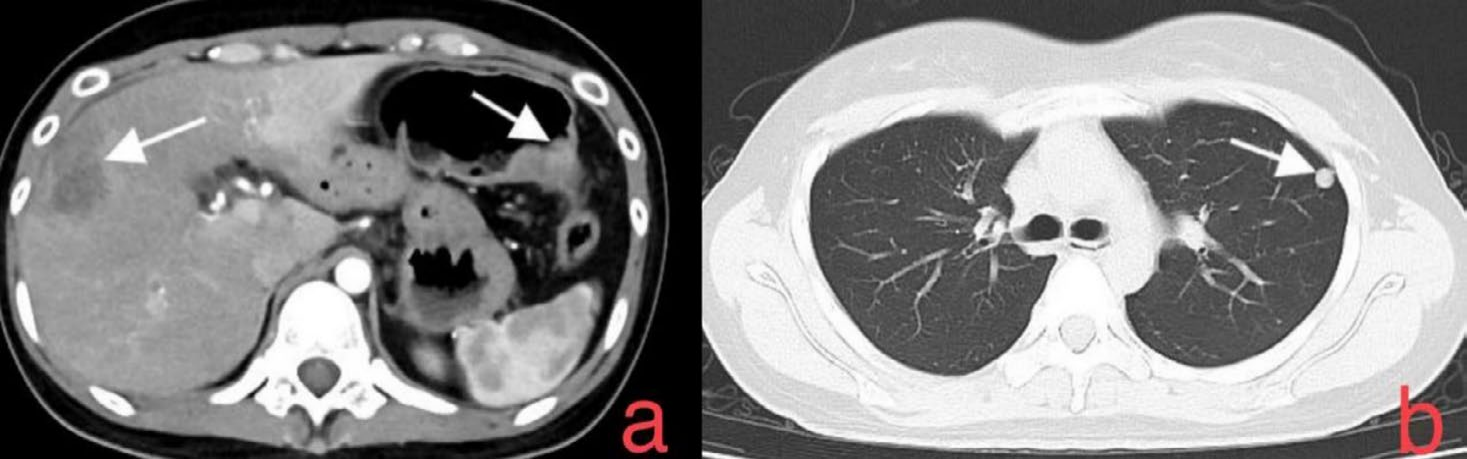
(a) Contrast‐enhanced abdominal CT scan showing a mass in the anterior segment of the right hepatic lobe (left arrow), exhibiting heterogeneous ring‐like enhancement and irregular wall thickening with focal luminal narrowing at the splenic flexure of the colon (right arrow), consistent with colorectal cancer and secondary incomplete colonic obstruction. (b) Chest CT scan showing a round mass in the apicoposterior segment of the left upper lobe (arrow), suggestive of metastatic carcinoma.
